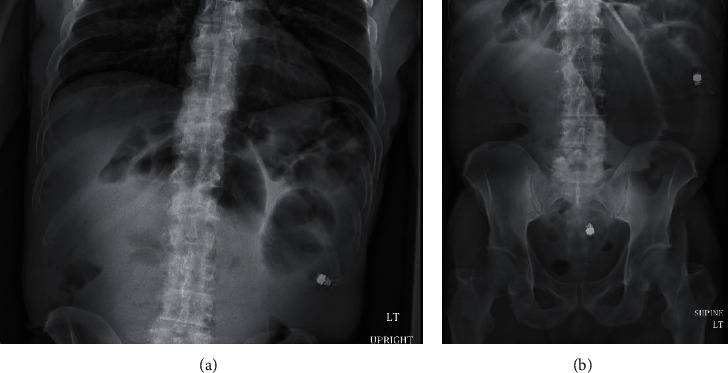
Upright (a) and supine (b) abdominal X-rays taken on admission showing dilated loops of small bowel in the central and left abdomen.
